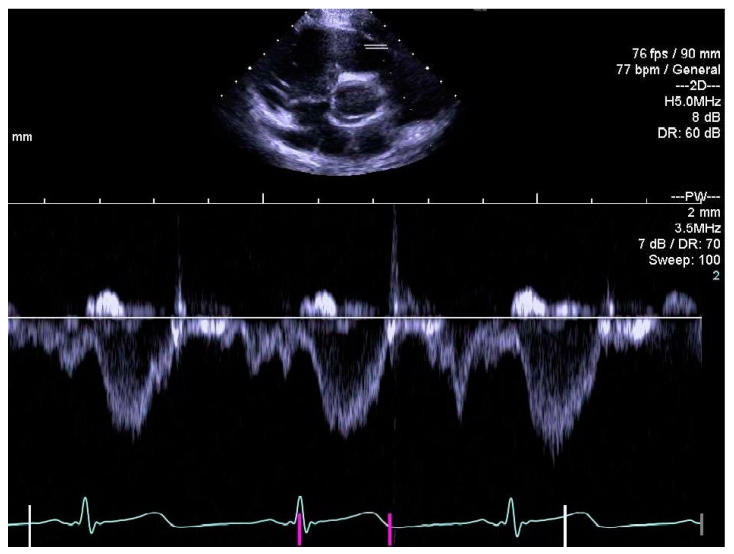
Pulsed wave Doppler of right ventricular outflow tract (RVOT) for measurement of velocity time integral, RVOTvti.
